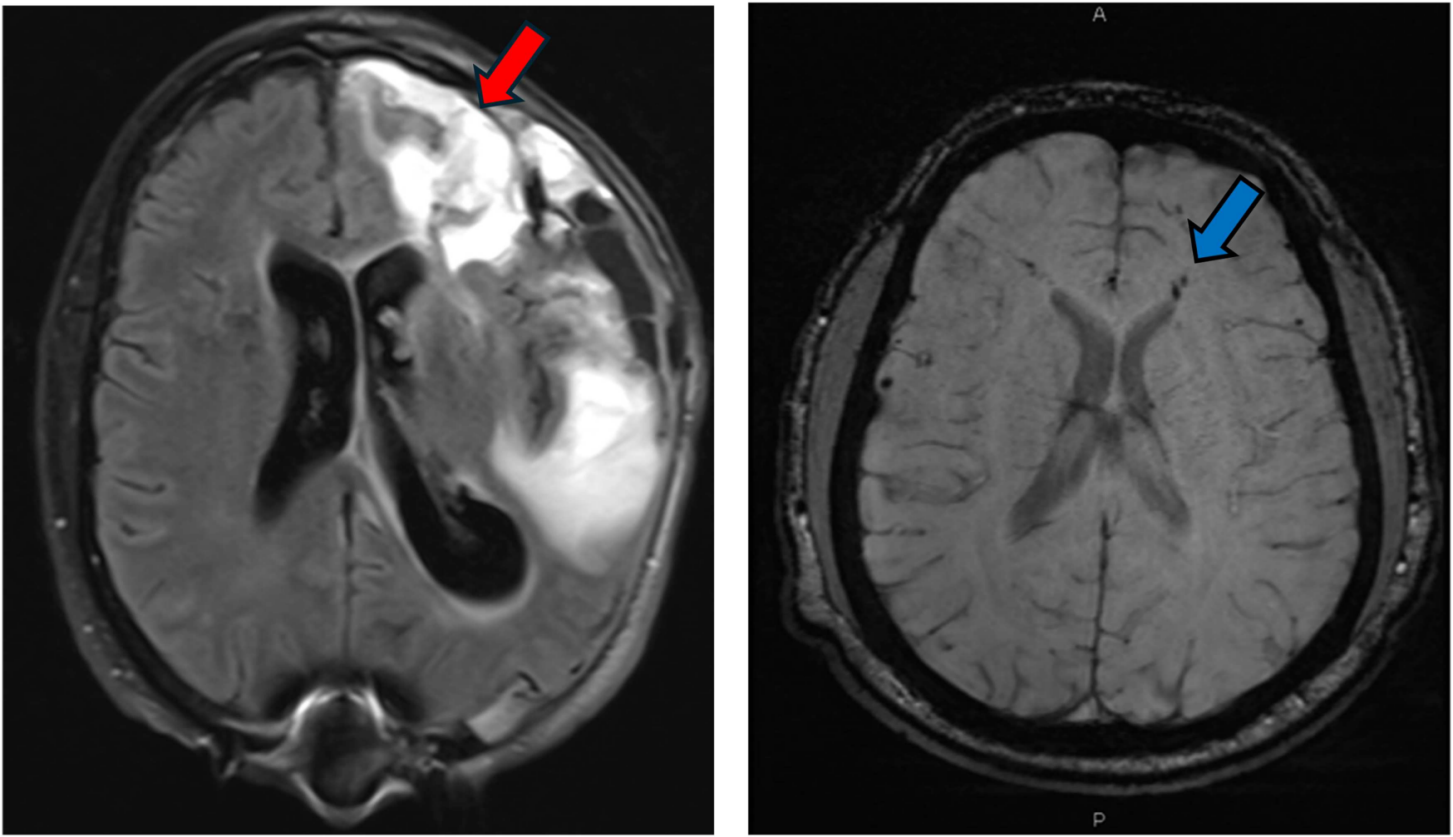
MRI FLAIR sequence demonstrating significant multicompartmental intraparenchymal hemorrhage (red arrow) post decompressive left hemicraniectomy for patient JP. MRI SWI sequence demonstrating multiple foci of intraparenchymal hemorrhages and diffuse axonal injury (DAI) for patient JS (blue arrow).
